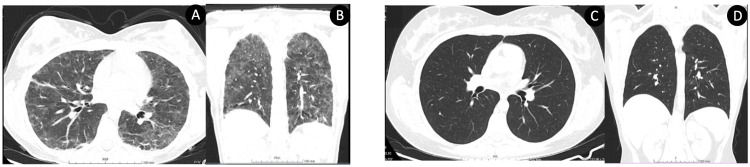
A and B. CT during the symptomatic phase; C and D. CT after treatment CT: computed tomography
